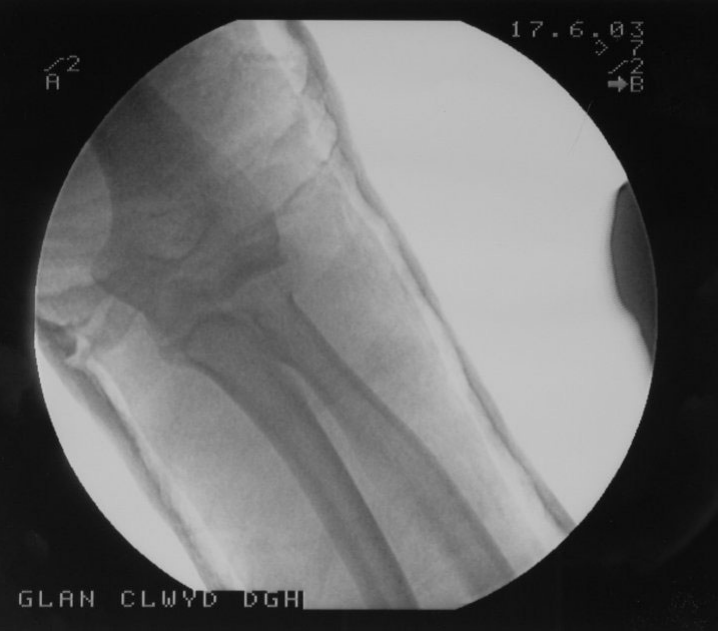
Post reduction anteroposterior radiograph of the elbow showing the radial head in reduced position.
